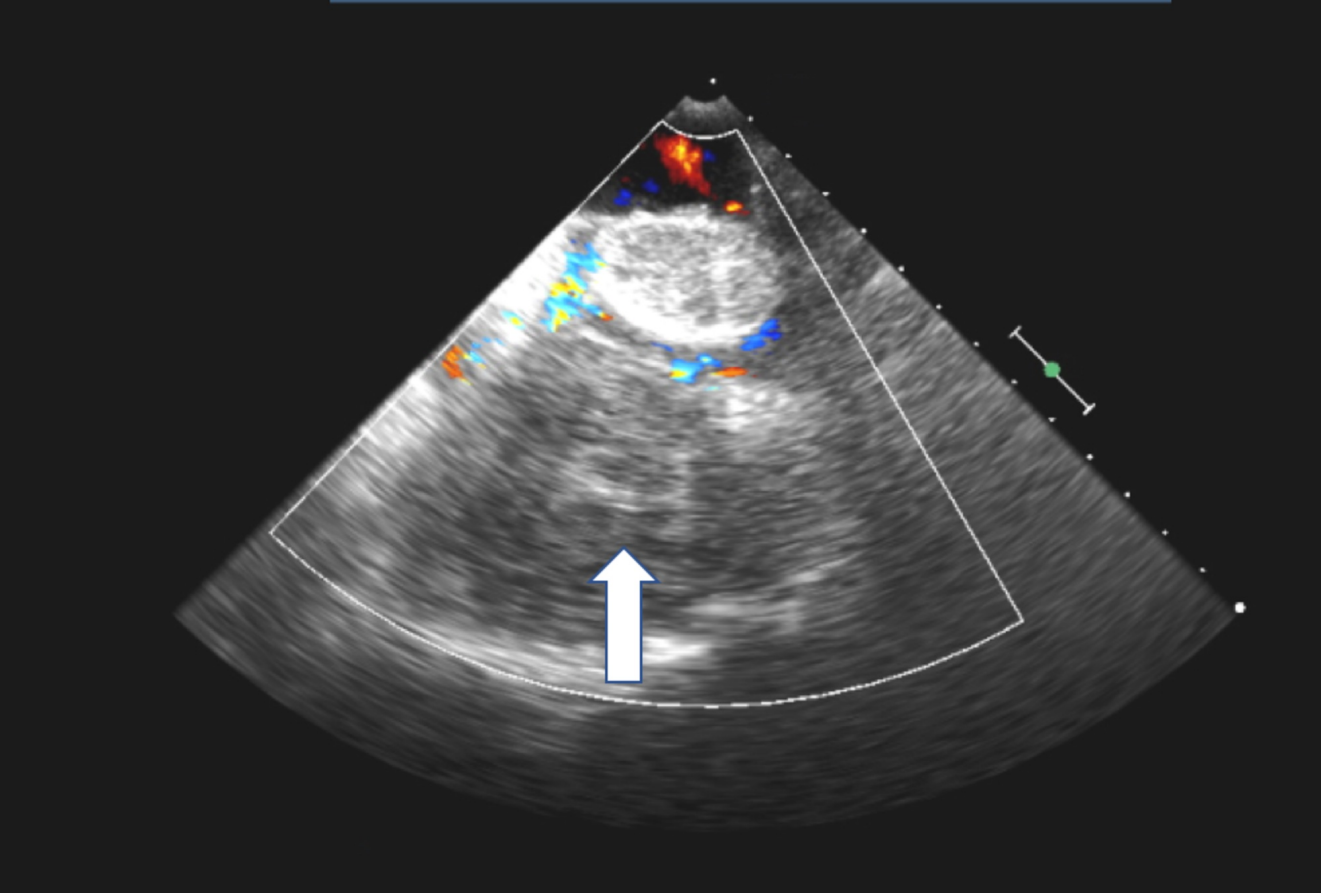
Pre-surgery transesophageal echocardiogram (TEE) image with color flow showing the pericardial hematoma Pre-surgery TEE showing pericardial hematoma (white arrow) compressing and nearly obliterating the right atrium. Image seen is with color flow.
